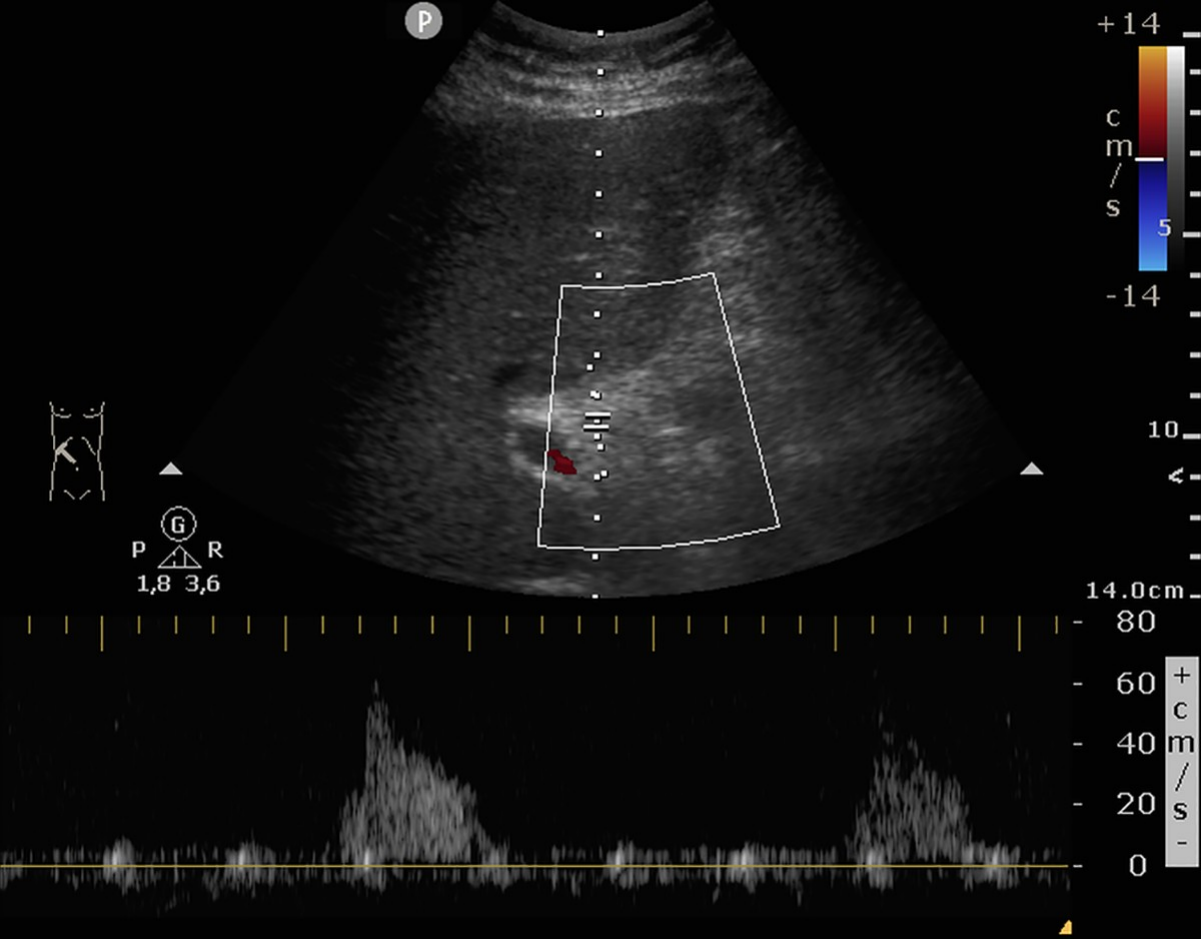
Vascular complications in the liver. In this patient with severe deterioration of liver function only residual arterial Doppler signal could be found in the porta hepatis. The patient died the same day.
